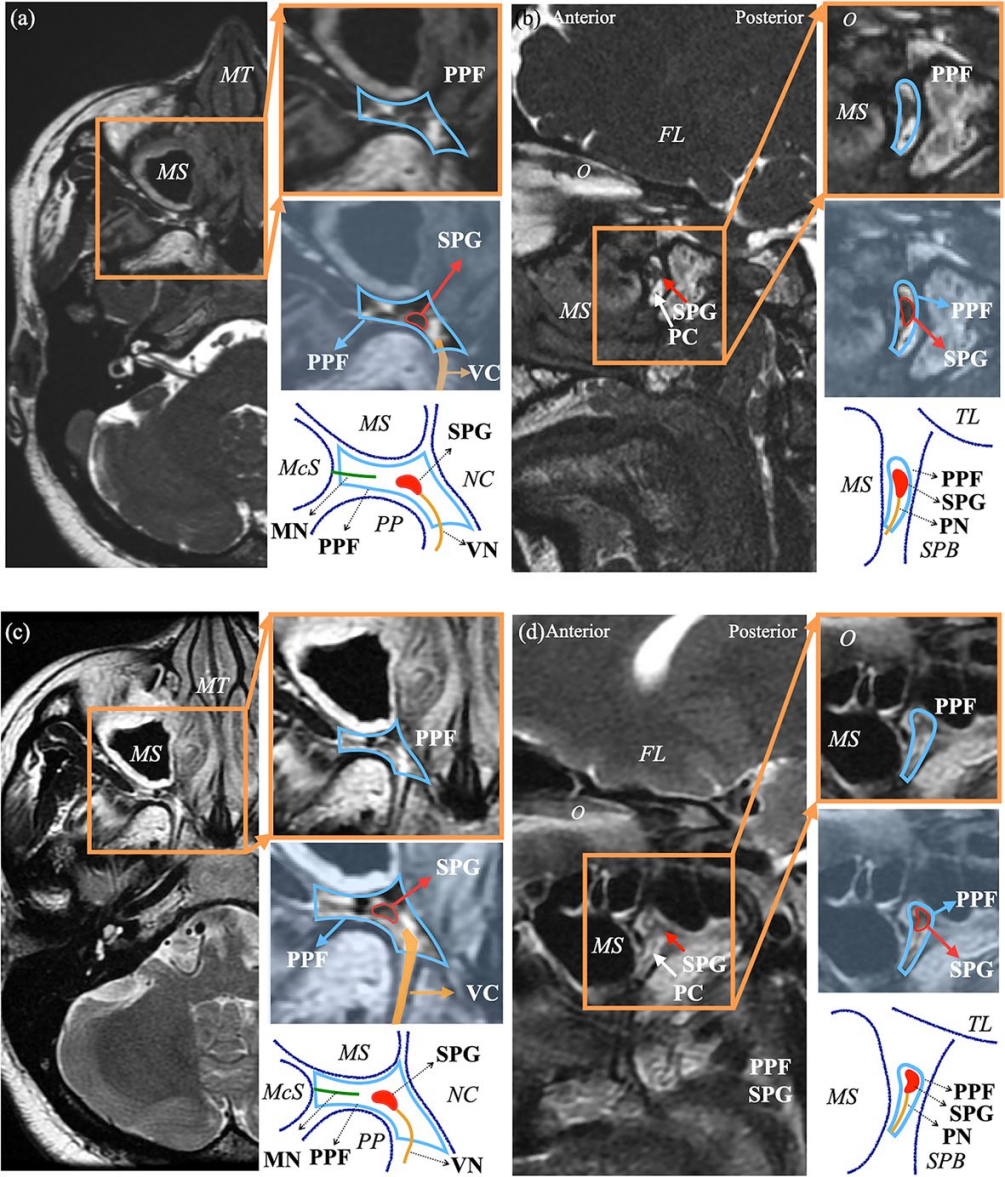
Visualization and measurement of the SPG. Axial (a) and sagittal (b) FIESTA MRI, axial (c) and sagittal (d) T2-weighted MRI depicting the sphenopalatine ganglion (SPG) (the red arrow is positioned in the center of the SPG, blue arrow is positioned at the pterygopalatine fossa [PPF]). The SPG has a crescent shape and intermediate signal intensity. The image surrounded by the orange rectangle is the ‘zoomed-in’ image. The light blue polygon depicts the border of the PPF. The oval red line is the border of the SPG. The dark blue line depicts the adjacent structure of PPF. FL cerebral frontal lobe, McS masticator space, MN maxillary nerve, MS maxillary sinus, MT middle nasal turbinate, NC nasal cavity, O orbit, PC palatine canal, PN palatine nerve, PPF pterygopalatine fossa, PP pterygoid process, SPB sphenoid bone, TL temporal lobe, VC vidian canal, VN vidian nerve
